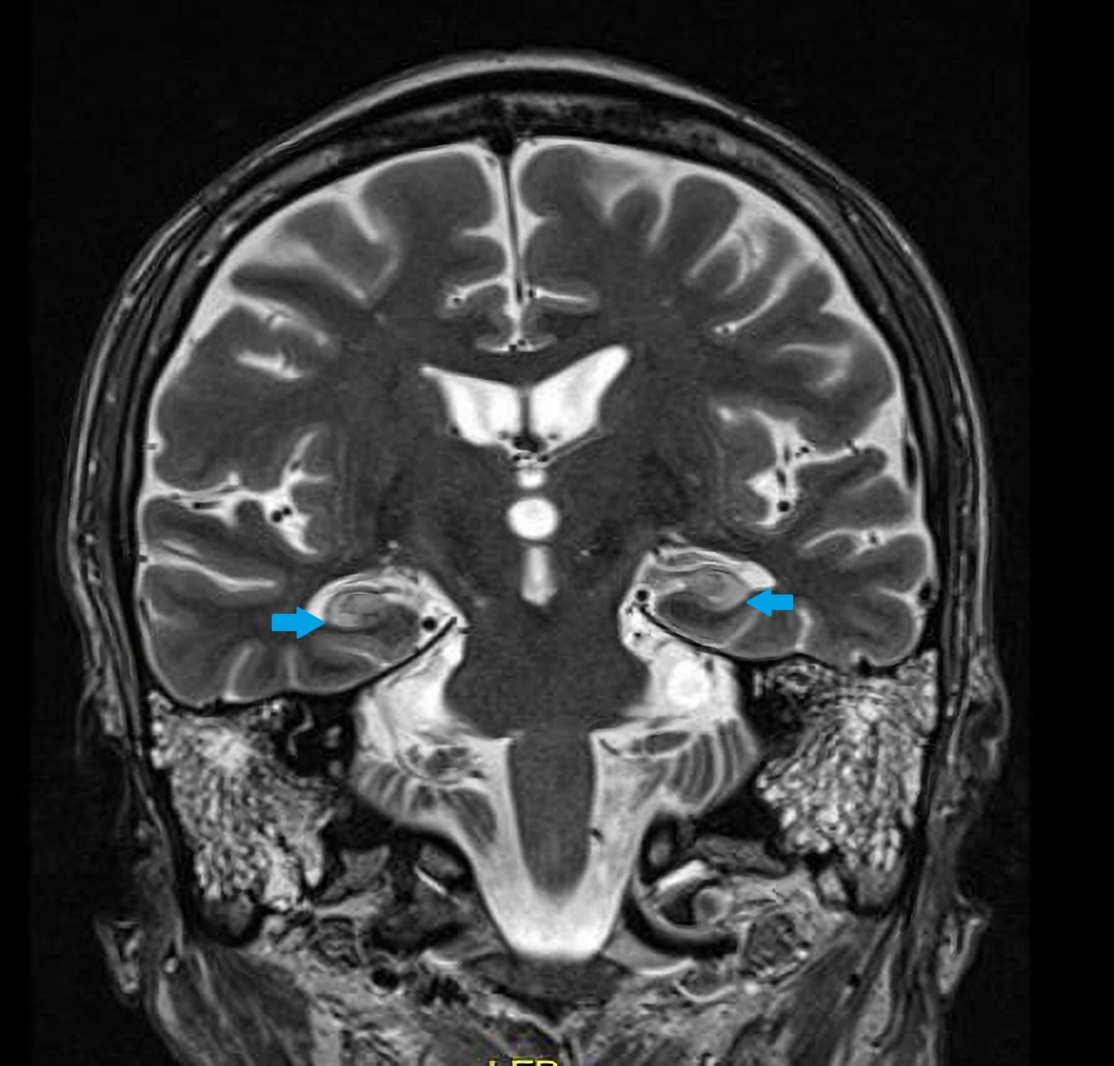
Magnetic resonance imaging (MRI) brain coronal T2 image demonstrating increased T2 signal in the bilateral hippocampi (blue arrows).
